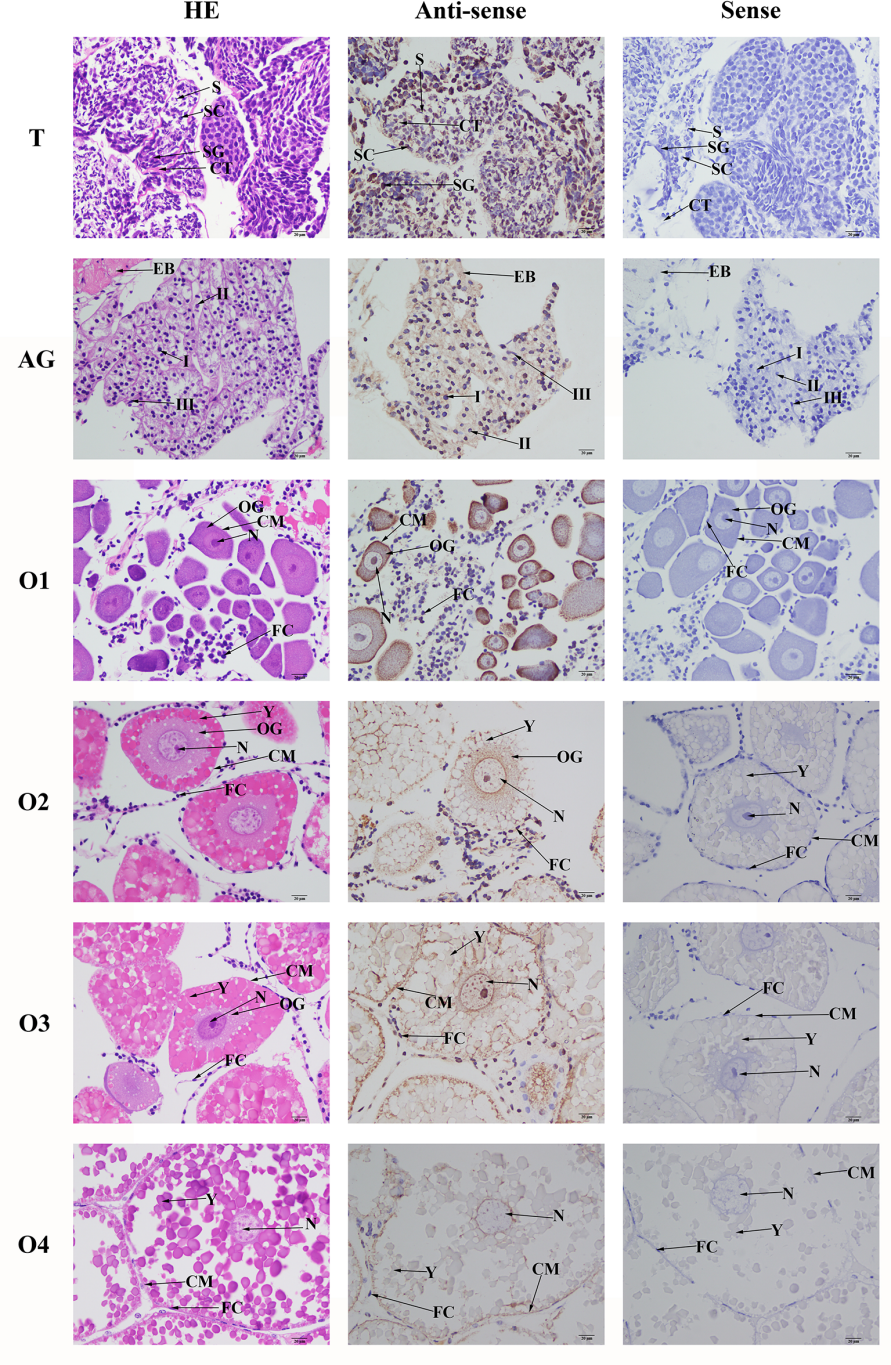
Location of *PDHE1* gene was detected in testis, androgenic gland and ovary of *M*. *nipponense* by using *in situ* hybridization. ST, seminiferous tubule; SG, Spermatogonia; SC, spermatocyte; S, sperm; CT, collecting tissue; E, wall epithelium; EM, eosinophilic matrix; VD, vas deferens; EB, ejaculatory bulb; OG, oogonium; OC, oocyte; CM, cytoplasmic membrane; N, nucleus; Y, yolk granule; FC, follicle membrane. Scale bars = 20 μm.
